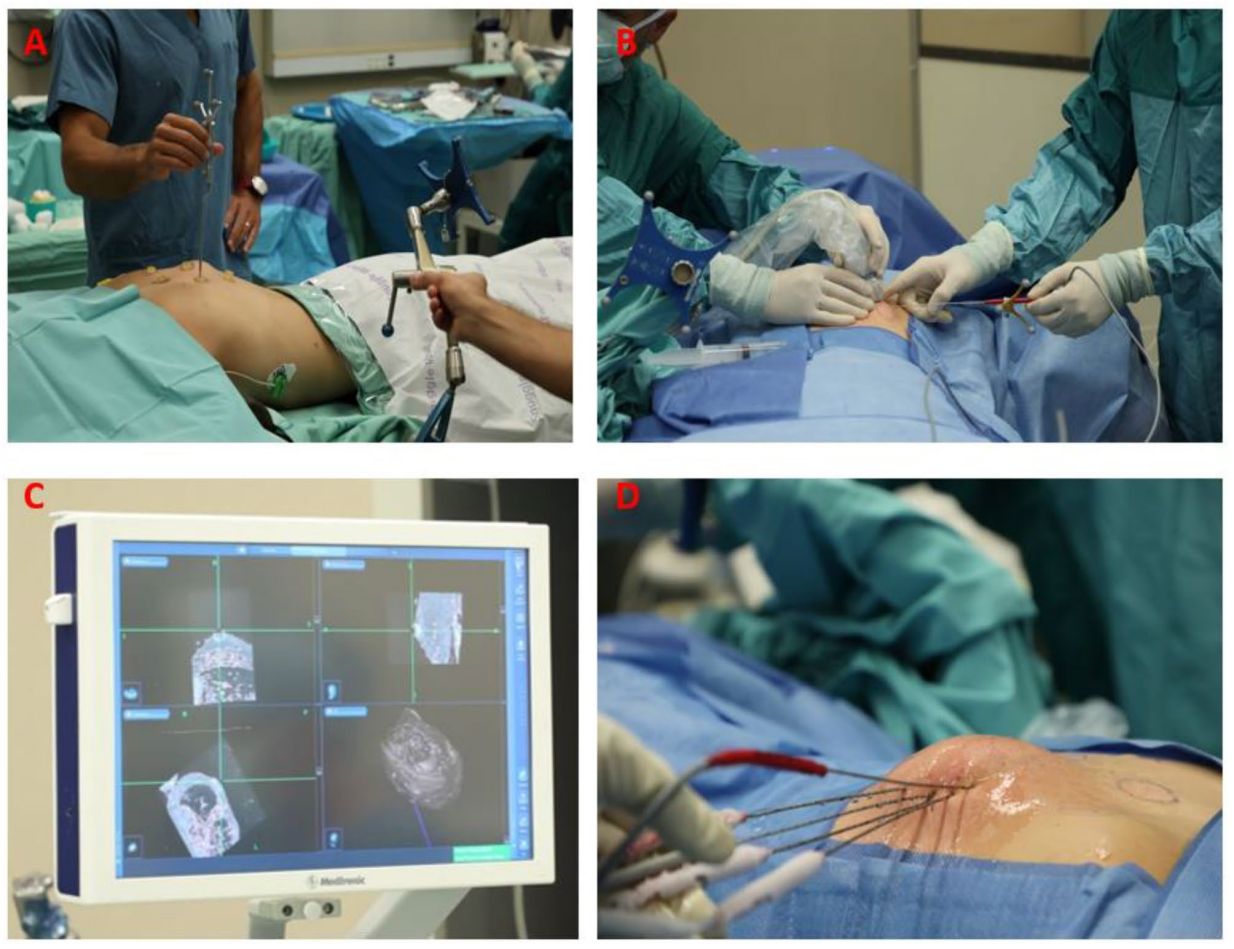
(A) Pointer registration of each fiducial marker. (B) The sure track system is attached to cryo-needle and calibrated. The needle can be inserted into the tumor using navigation according to pre-operative 3D planning. Ultrasonography was used to verify position. (C) Stealth station MRI screen navigation shows bright and dark areas, indicating desmoid tumor viability. (D) Cryo-needles inserted according to the preoperative plan, using warm saline to protect the skin. A thermometer guide needle was also used to monitor and maintain core tumor temperature of below − 40 degrees Celsius
